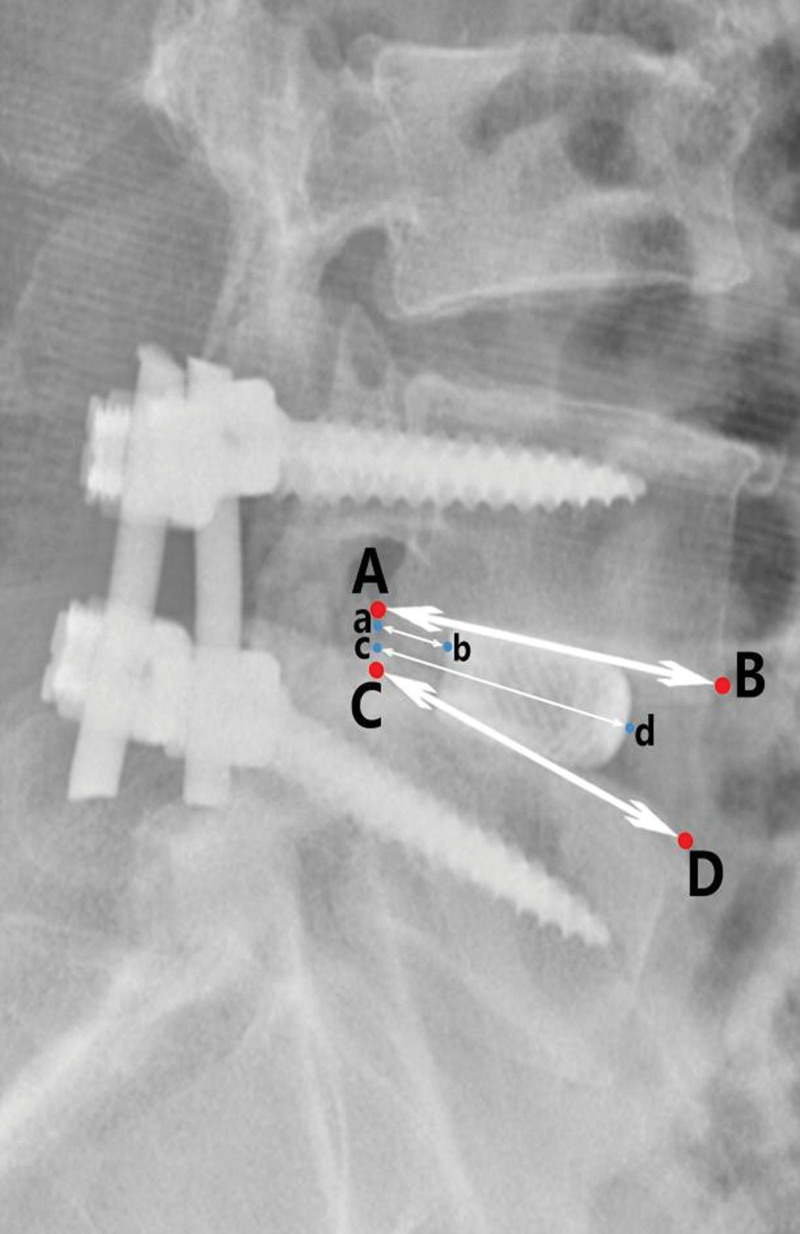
Lateral X-ray of lumbar vertebra. Central ratio = (ab + cd)/(AB + CD)*100%.
